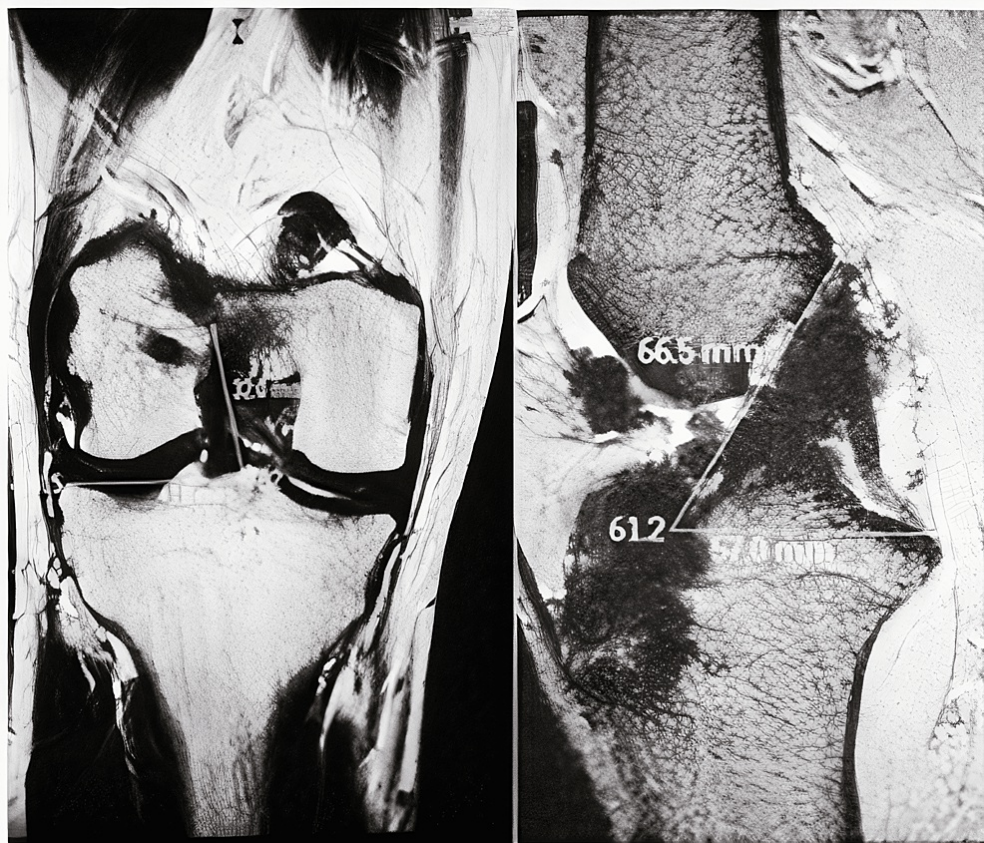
MRI showing the knee with double-bundle reconstruction
